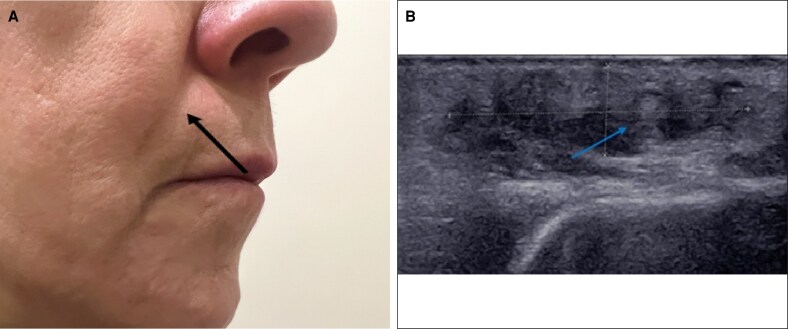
(A) Clinical and (B) ultrasound view of a 48-year-old female with a history of polycaprolactone injection in the bilateral nasolabial folds (black arrow in A). The ultrasound axial view shows a 32 × 9 mm ill-defined hypoechoic nodule with irregular borders and some echogenic foci compatible with granuloma (blue arrow in B).
